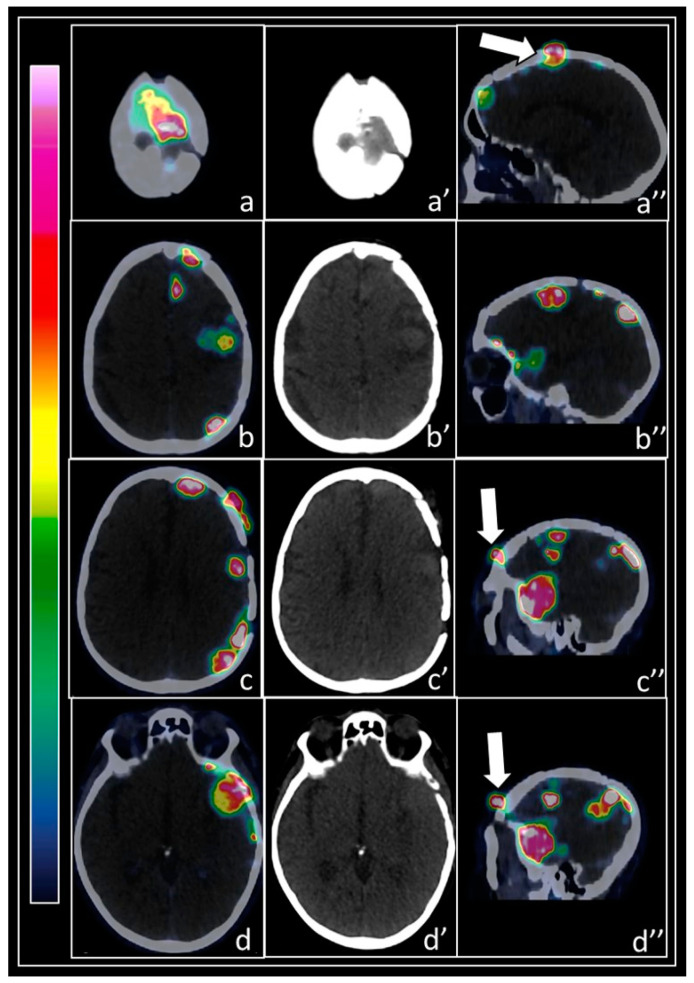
A 70-year-old patient with multiple meningiomatosis, already submitted to several consecutive interventions of surgical excision in the left-brain hemisphere and two treatments with cyberknife stereotactic radiosurgery for recurrences, was examined by brain PET/CT with ^68^Ga-DOTATOC for an evaluation of tumor extent as suggested by Laudicella et al. [34]. PET/CT displayed multiple foci of pathologic tracer uptake in the left-brain hemisphere, particularly in the cranial vertex, as shown in the axial PET/CT (a) and CT (a’) views, corresponding to a tracer-avid lesion protruding into the scalp, also evident in the correlative sagittal PET/CT view (a’’, arrow). The patient clinically presented skull deformation in the vertex of the skull. Other tracer-avid parafalcal and subcortical lesions were also detected, shown in the axial PET/CT (b) and CT (b’) views and correlative sagittal PET/CT view (b’’). Moreover, multiple cortical tracer-avid lesions were observed in the left-brain hemisphere, as evident in axial PET/CT (c) and CT (c’) details. Some of these lesions were protruding into the subcutaneous tissues in the left frontal region, as depicted in the sagittal PET/CT view (c’’, arrow). Finally, intense ^68^Ga-DOTATOC uptake was recorded in a slightly hyperdense area in the left temporal region, invading subcutaneous soft tissues in the ipsilateral scalp. This finding is summarized in the axial PET/CT (d) and CT (d’) views and sagittal PET/CT view (d’’, arrow).
